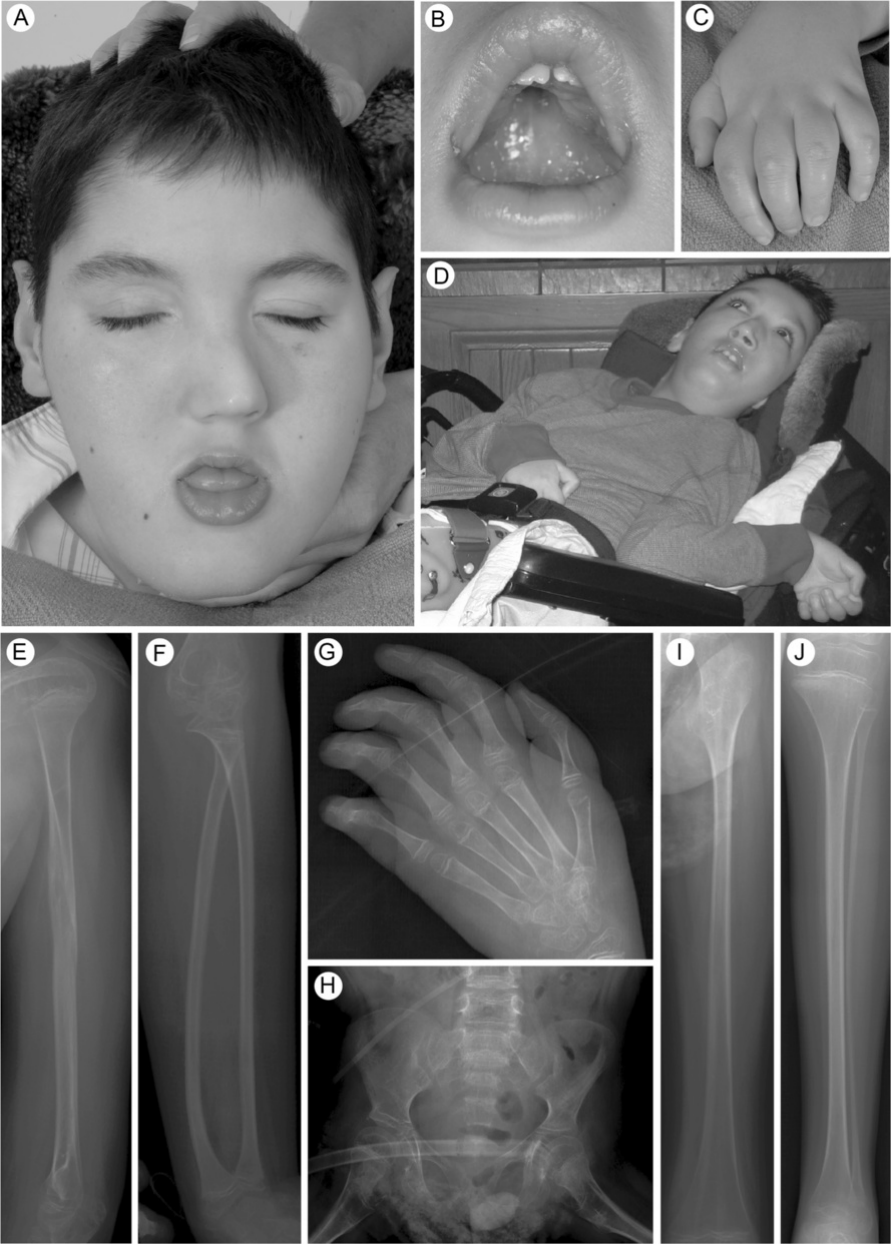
Clinical and radiographic features. A. Face of patient II-1. B. Face of patient II-3. C. Palate of patient II-1. D. Hands of patient II-3. E-J. Skeletal radiographs of Patient II-1 showing the left humerus (E), left forearm (F), left hand (G), pelvis (H), left femur (I) and left lower leg (J). Note the gracile bones and undermineralization as well as the healing humeral fracture (E).
